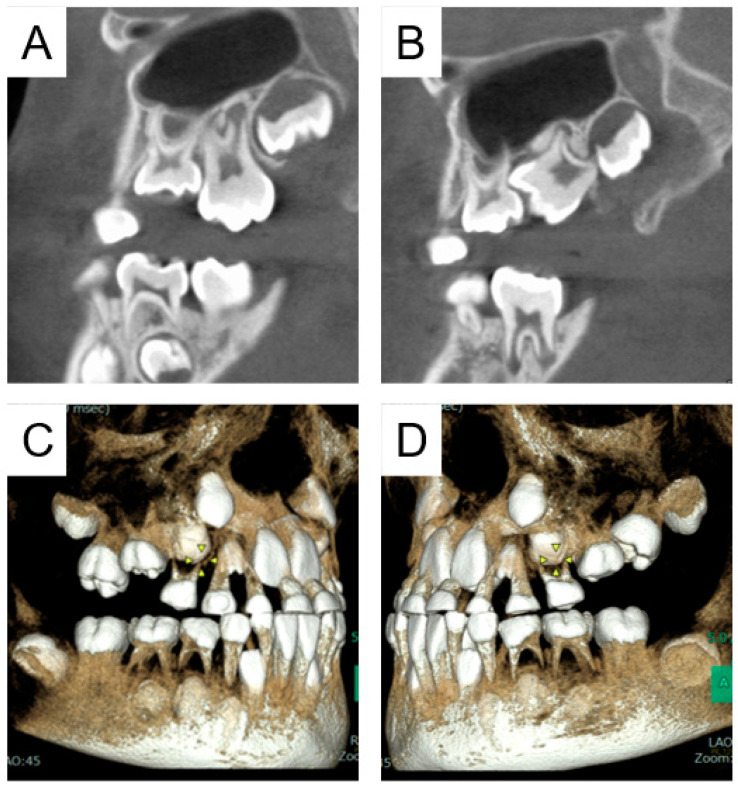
Cone-beam computed tomography images of Case 1 obtained at 7 years 0 months showing eruption disturbance of the maxillary left permanent first molar. Sagittal section at maxillary right first molar (A) and left first molar (B). Three-dimensional construction images at right (C) and left (D).
